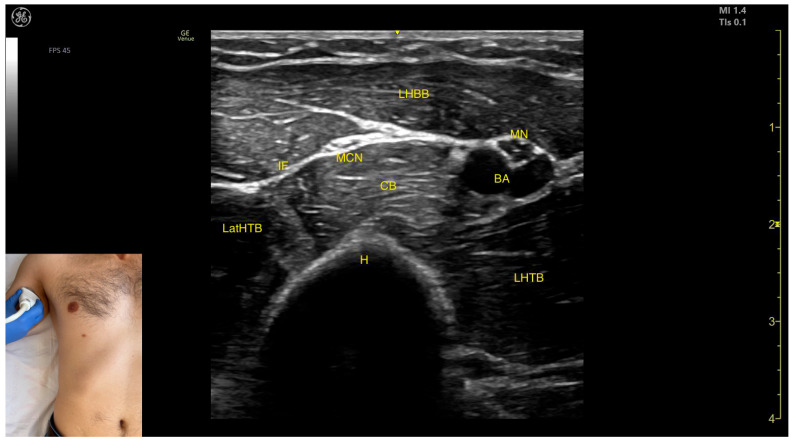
Ultrasound anatomy of the long head biceps brachii (LHBB) with key landmarks: LHBB—long head biceps brachii; IF—intermuscular fascia; MCN—musculocutaneous nerve; CB—coracobrachialis; MN—median nerve; BA—brachial artery; LatHTB—lateral head triceps brachii; H—humerus; and LHTB—long head triceps brachii.
